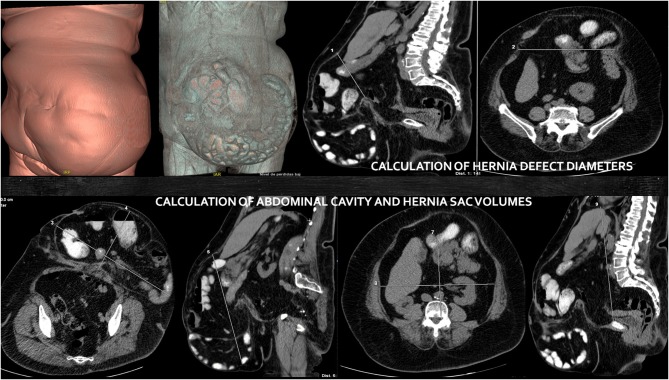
Volumetric CT images before botulinum toxin and progressive pneumoperitoneum application. Calculation of hernia sac and abdominal cavity volumes and hernia defect diameters.
